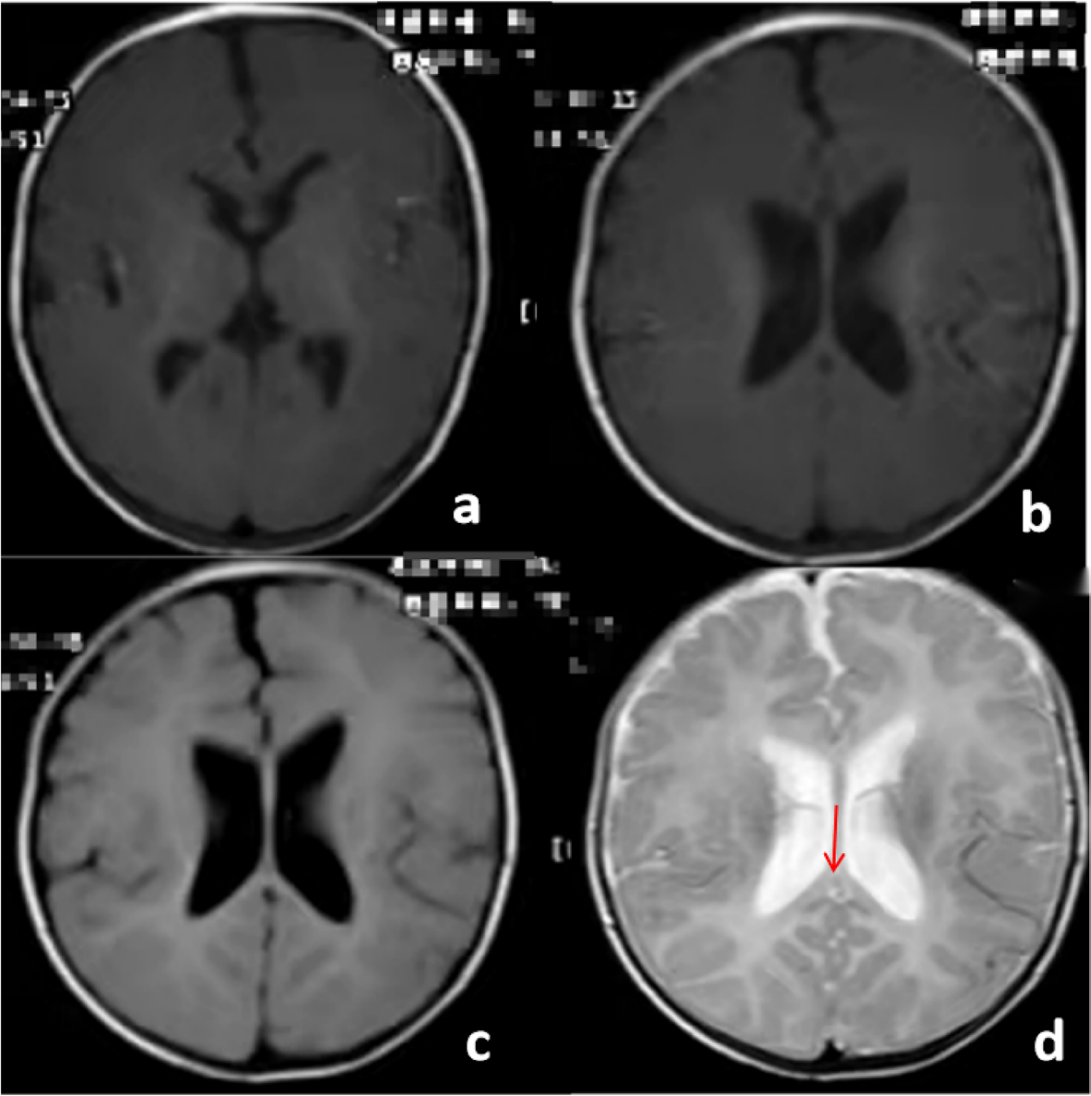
Brain MRI revealed dysplasia of the corpus callosum and bilateral ventricle dilatation (a-d)
